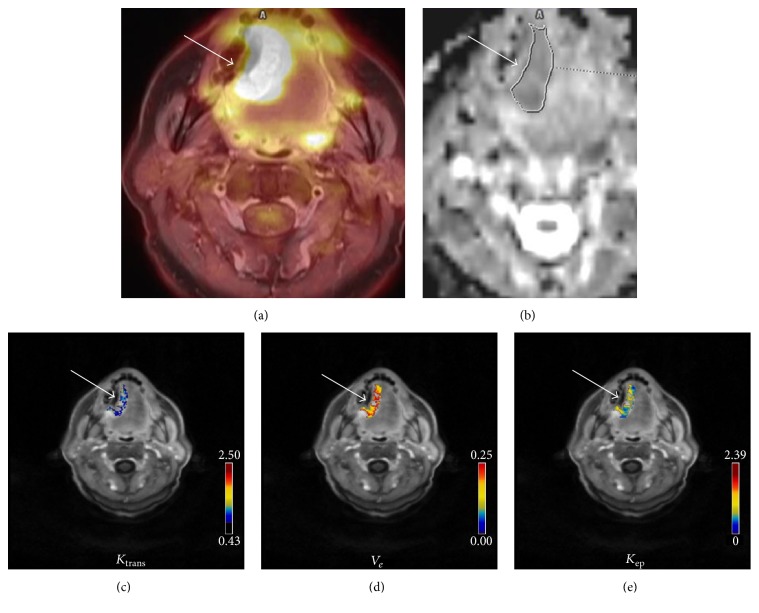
Imaging findings in a 58-year-old man with squamous cell carcinoma of the tongue (G1, T4 N2 M0). (a) ^18^F-FDG-PET imaging (fused image) showing a right sided large lesion of the tongue; SUV_max_ = 24.11. (b) ADC map. The ADC values (×10^−3^ mm^2^s^−1^) of the lesion are as follows: ADC_min_ = 0.68, ADC_mean_ = 0.97, and ADC_max_ = 2.1. (c–e) DCE imaging findings. Estimated DCE parameters are as follows: *K*_trans_ = 0.53 min^−1^ (c), *V*_*e*_ = 0.68% (d), and *K*_ep_ = 0.75 min^−1^ (e).
